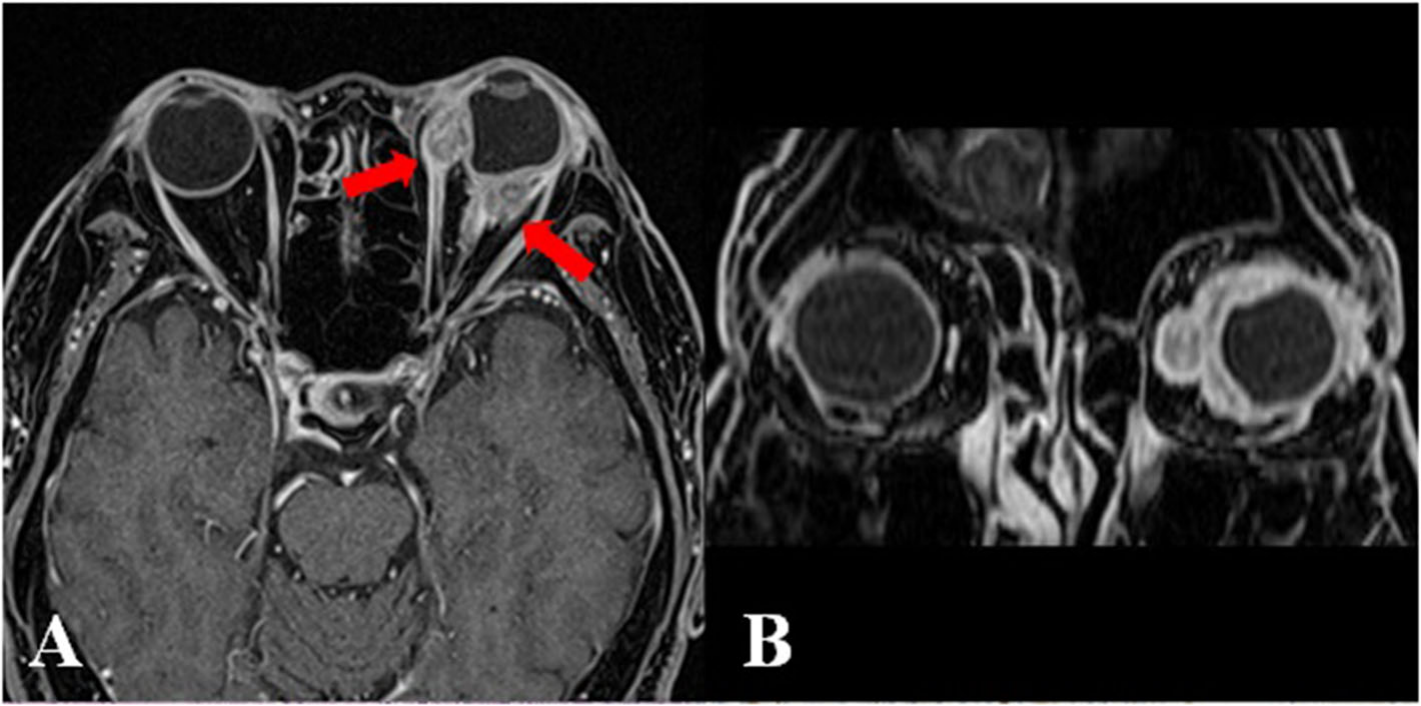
Orbit MRI demonstrated enhancing soft tissue encasing the left globe (a & b) with encasement of left medial rectus muscle and optic nerve (red arrows)
